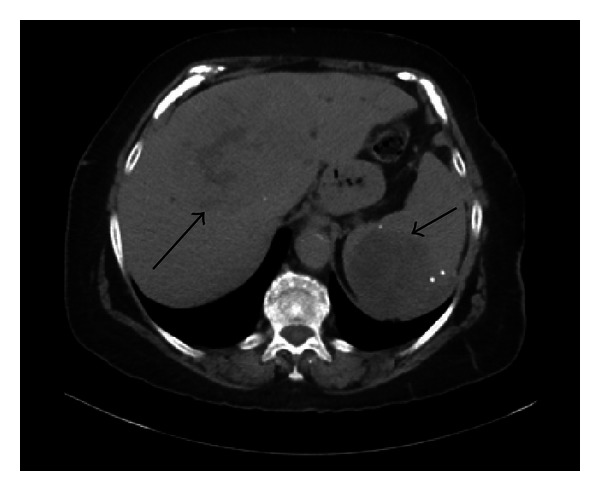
Case 2—CT scan abdomen pelvis with infiltrating ill-defined liver mass involving the portal confluence and spleen mass (black arrows).
